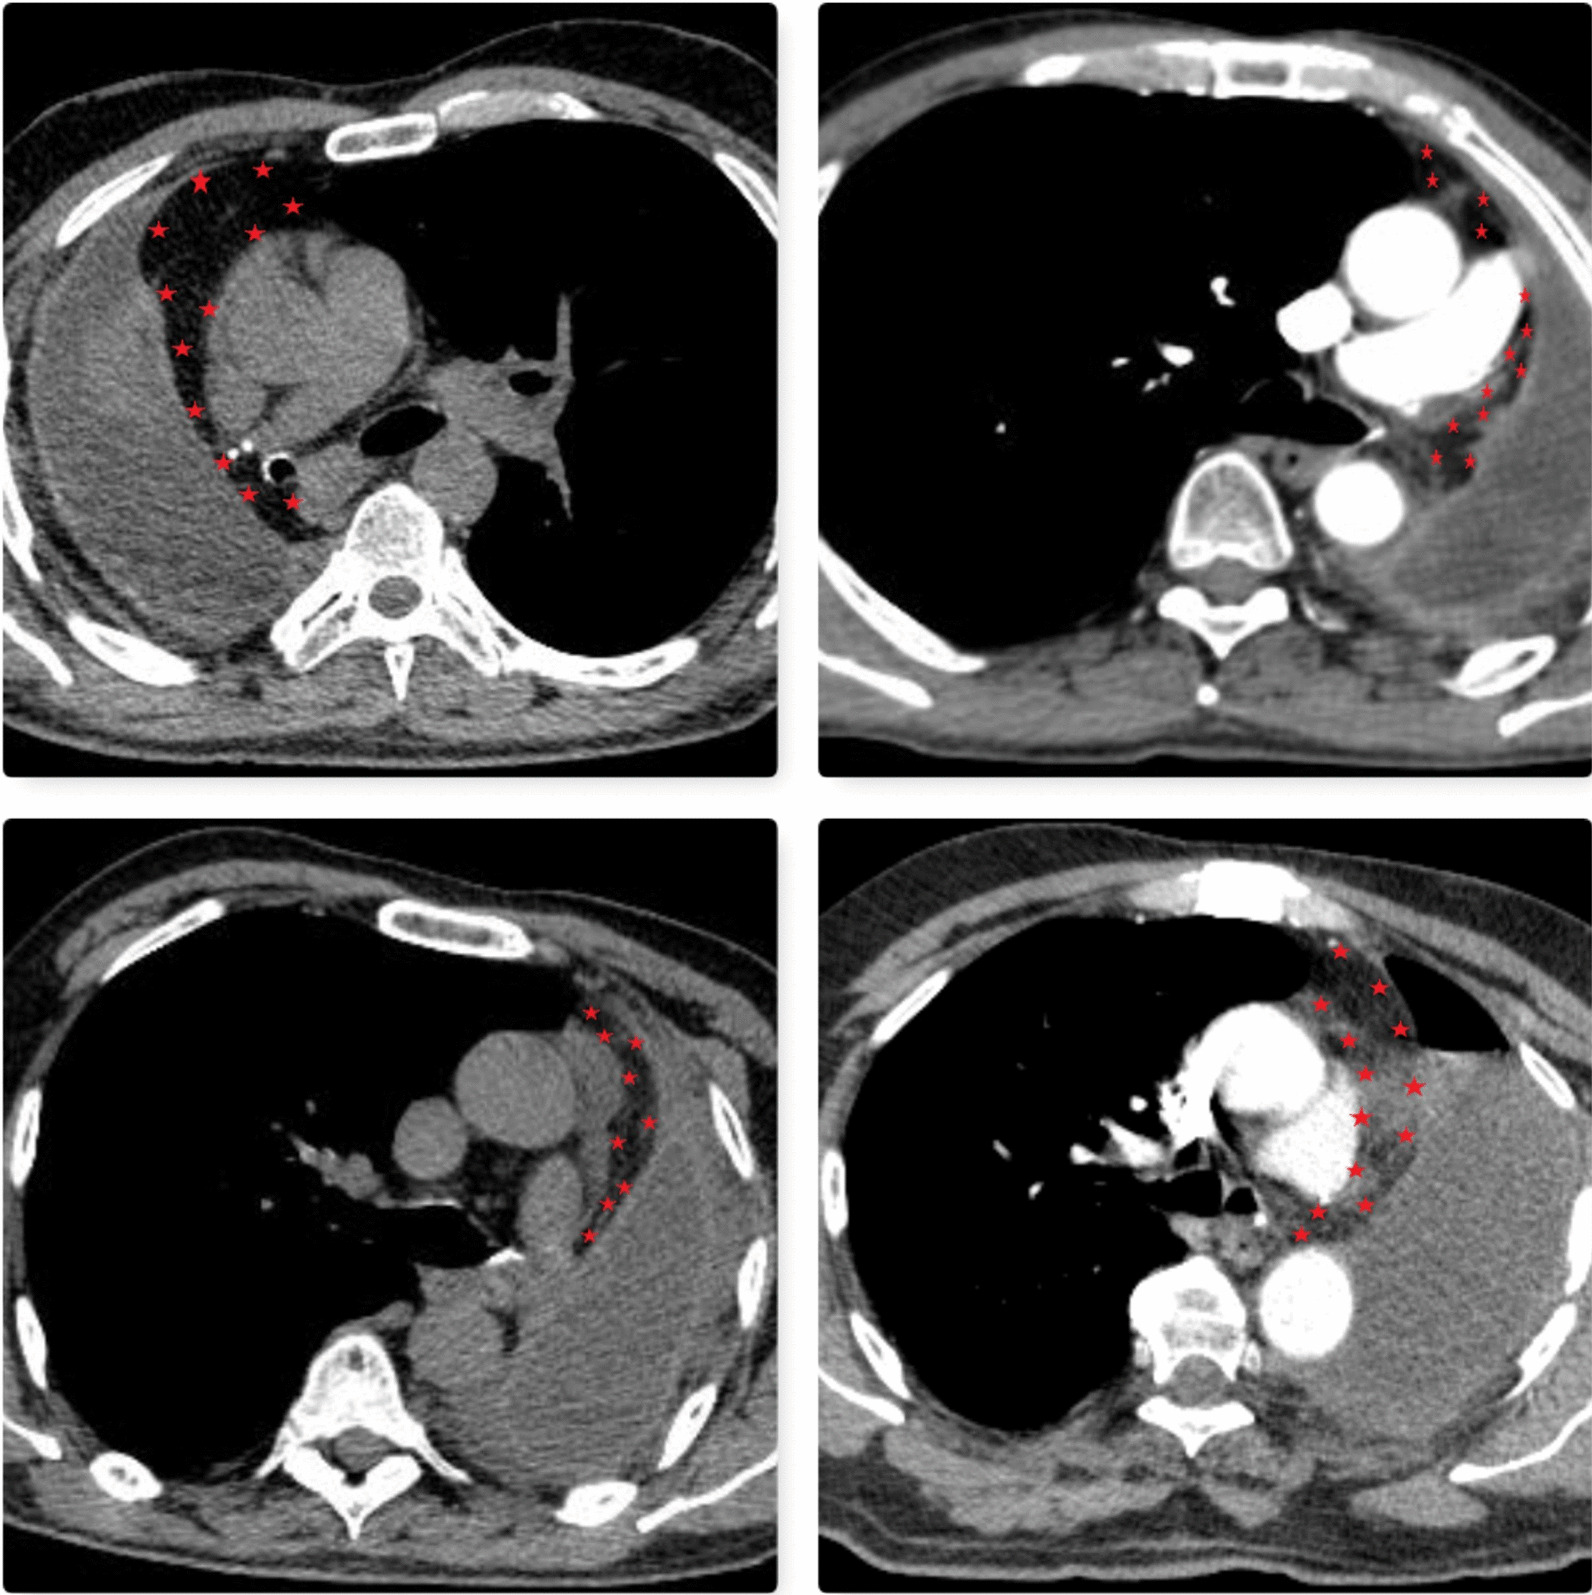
TPFF could be seen in thorax CT even after 6–12 months after operation (asterisks)
